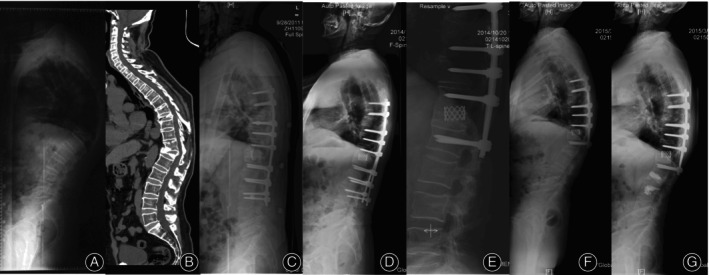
A 70‐year‐old man present with back pain, visual analogue scale (VAS) 8. (A) Lateral x‐ray showed thoracolumbar hyper‐kyphosis arising from T12 fracture, global kyphosis (GK) was 98°, thoracolumbar kyphosis (TLK) was 42.9°, sagittal stable vertebrae (SSV) located at L3. (B) Computed tomography revealed T8 and T12 fracture. (C) Postoperative lateral x‐ray showed T6‐L3 fixation, GK and TLK was 37.4° and 4.9°, respectively. (D) Pull‐out of L2 and L3 screws were detected at 3‐years' follow‐up. (E) After revision surgery, L2 and L3 screws were removed. (F) Five months later, the patient had a L2 fracture after falling. (G) Reoperated with vertebroplasty in L2 and L3, GK and TLK was 52.9° and 15.8°, respectively.
